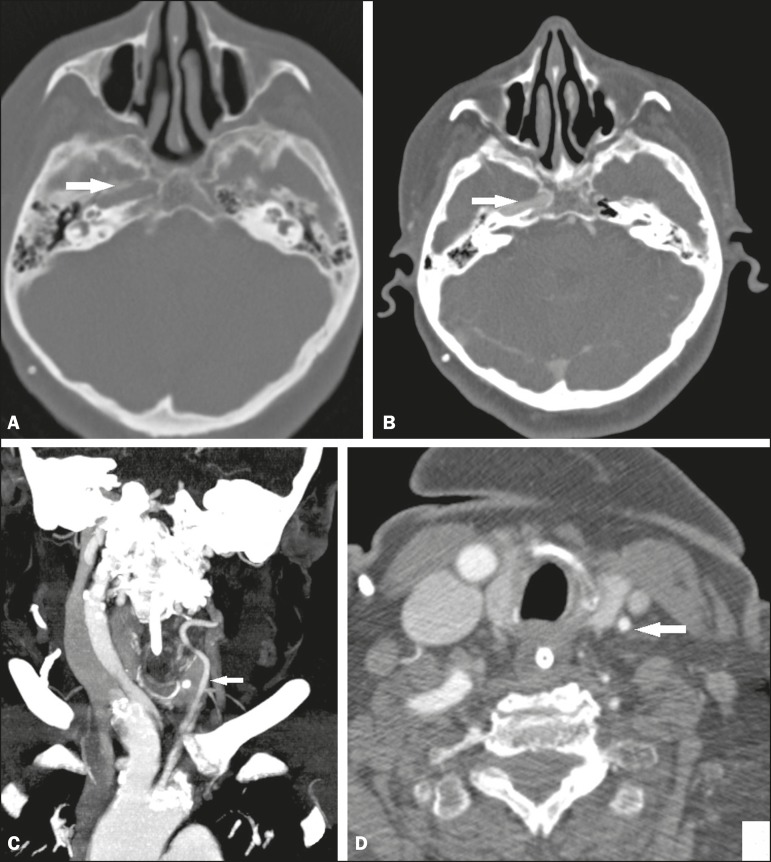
Axial CT scan of the brain (A,B) showing a normal right carotid
